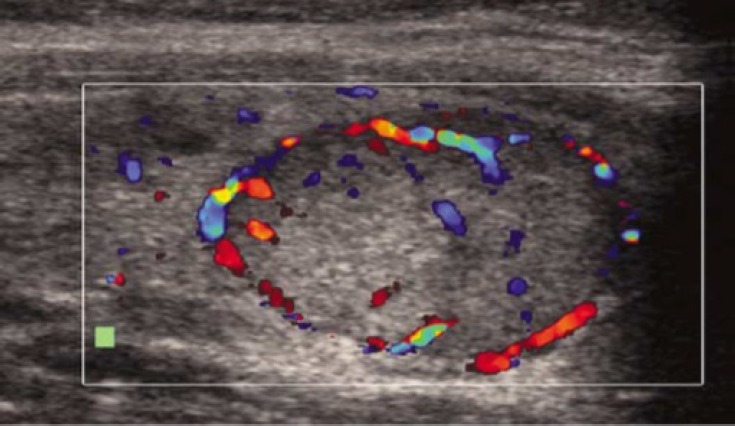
Preference perinodular hypervascularity with exclusively perinodular blood flow on color Doppler sonography as the most common finding in benign nodules
